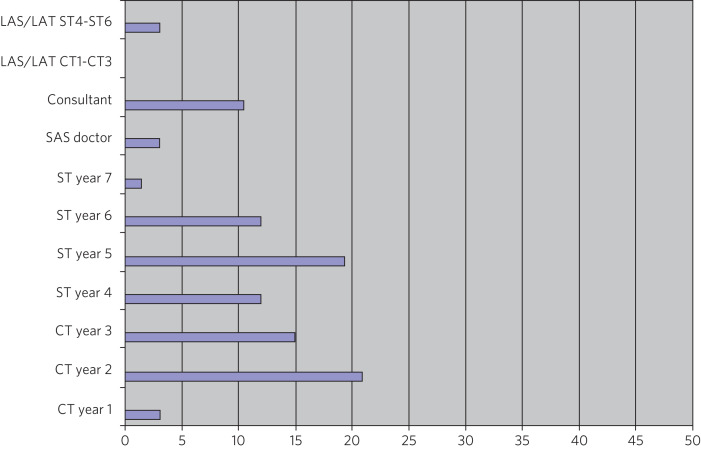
Grade breakdown of participants, *n* = 62. LAS, Locum Approved Service; LAT, Locum Approved Training; SAS, Specialty and Associate Specialist; ST, Senior Trainee; CT, Core Trainee.
